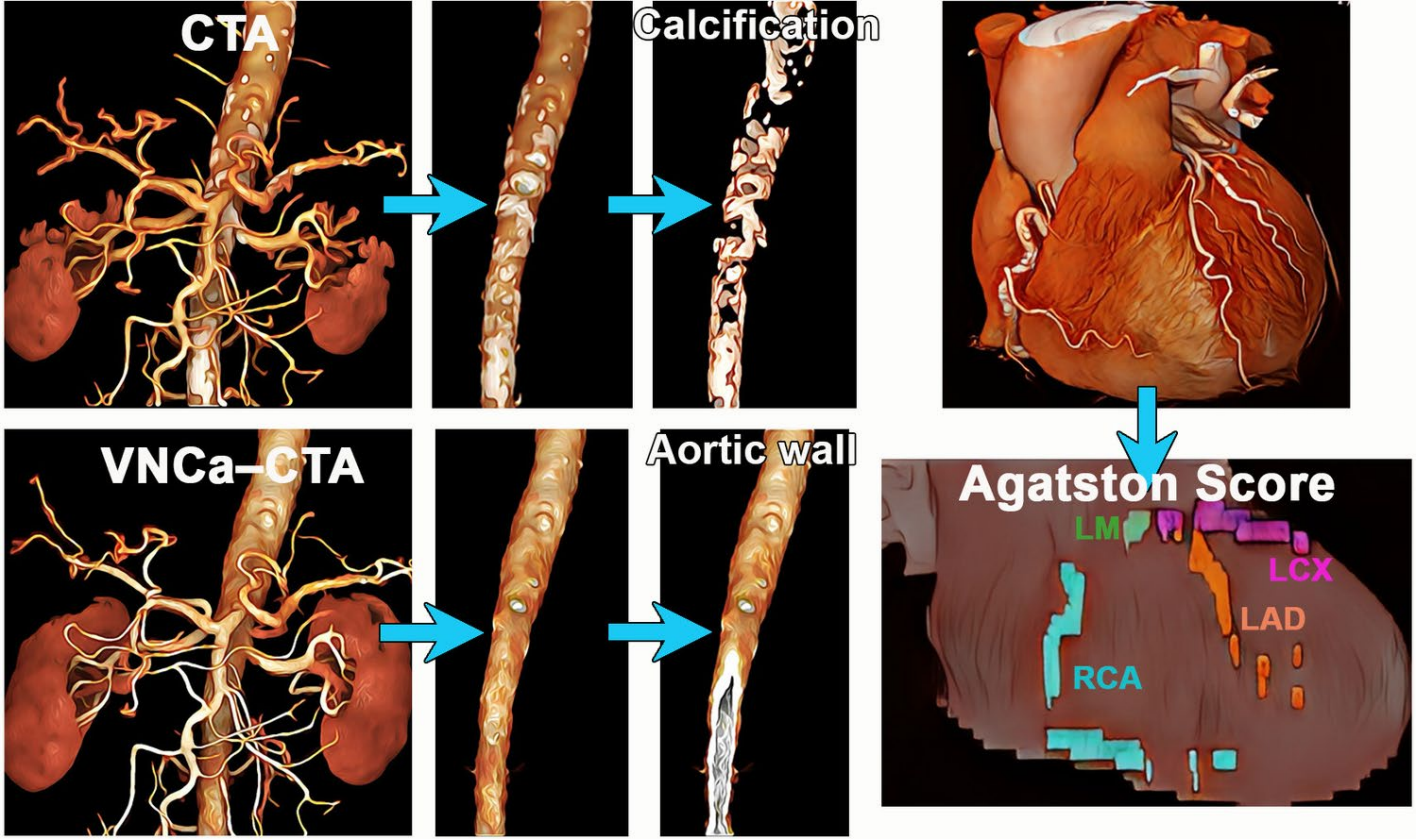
Research design. Calcification in the abdominal aortic wall was extracted from 3D CTA images and aortic calcification volume (ACV) was measured. In addition, the aortic wall was constructed from 3D CTA images with VNCa applied and aortic wall volume (AWV) was measured. Percentage calcification volume (PCV) is the ratio of ACV to AWV. Coronary artery calcification was quantified using a dedicated workstation to calculate the Agatston score. Using the Agatston score indicators as outcomes, we investigated the relationship between ACV and PCV and attempted to define the severity of abdominal atherosclerosis. CTA = CT angiography; VNCa = virtual non-calcium
